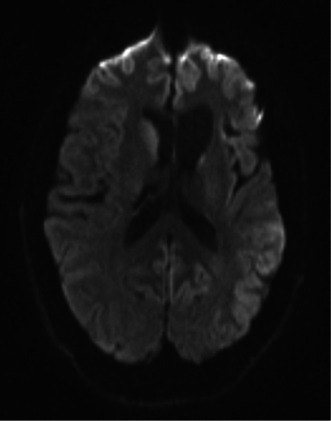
FIGURE 3 MRI brain demonstrated diffusion restriction in the bilateral basal ganglia with multifocal cortical ribboning, more prominent on the left.
